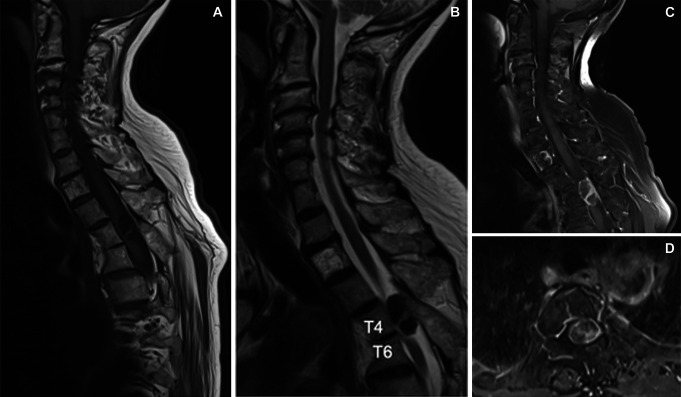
The neoplasm was hypointense on A, T1-weighted and B, T2-weighted images and peripherally enhanced with gadolinium on C, a sagittal and D, axial T1-weighted image.
